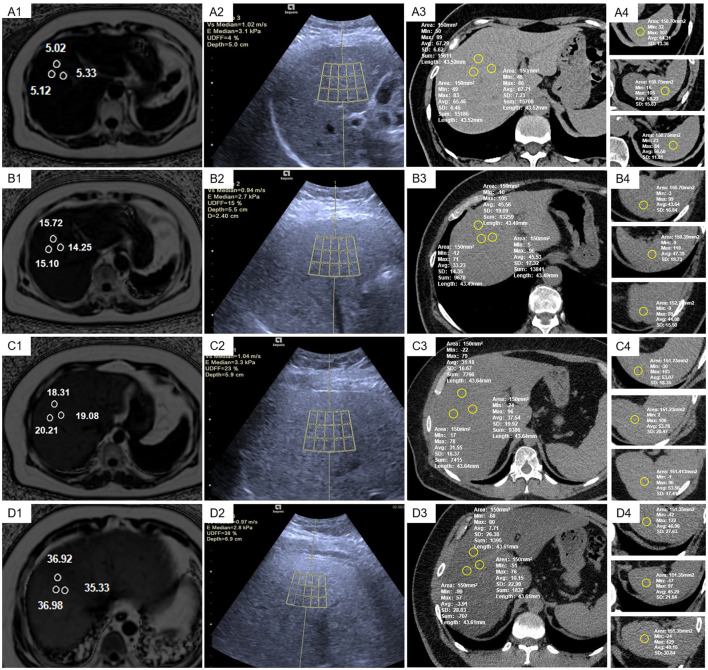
Representative images of MRI-PDFF, UDFF, CT(L/S) in four subjects. (A1–A4) A 61-year-old female patient presented with 5.12% MRI-PDFF, 4% UDFF, 1.1 CT(L/S), corresponding to S0; (B1–B4) a 67-year-old female patient presented with: 15.10% MRI-PDFF, 15% UDFF, 0.9 CT(L/S), representing S1; (C1–C4) a 61-year-old female patient presented with: 19.08% MRI-PDFF, 23% UDFF, 0.6 CT(L/S), representing S2; (D1–D4) a 37-year-old male patient presented with: 36.92% MRI-PDFF, 38% UDFF, 0.1 CT(L/S), representing S3. MRI-PDFF, magnetic resonance imaging-proton density fat fraction; UDFF, ultrasound-derived fat fraction; CT(L/S), CT liver-to-spleen attenuation ratio.
